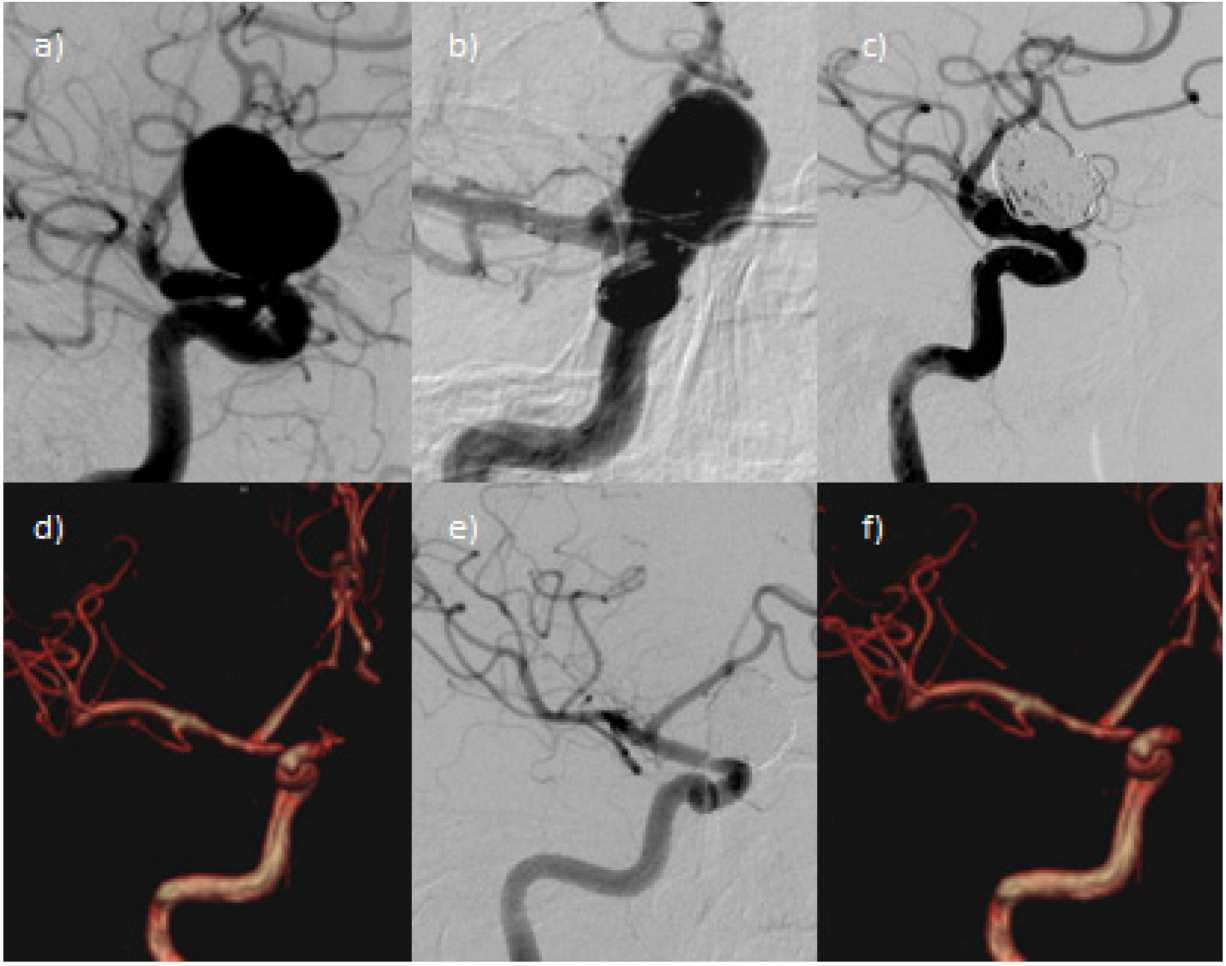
Unruptured supraclinoid ICA aneurysm treated by SAC with neuroform atlas stent and hydroSoft coils. a) DSA shows the aneurysm at the supraclinoid segment of right internal carotid artery (ICA) (max. width 18 mm); b) Angiographic vision of the Neuroform Atlas stent deployed between the M1-segment of the middle cerebral artery (MCA) and homolateral ICA, covering the aneurysmal neck. A microcatheter is positioned in the aneurysmal sac. c) The final angiogram shows a minimal neck remnant (RROC 2). d) The 3-months follow-up MRI confirms the RROC 2. e) The 6-months DSA control demonstrates complete occlusion of the aneurysmal sac (RROC 1); f) The 12-months MRI follow-up confirms RROC 1.
